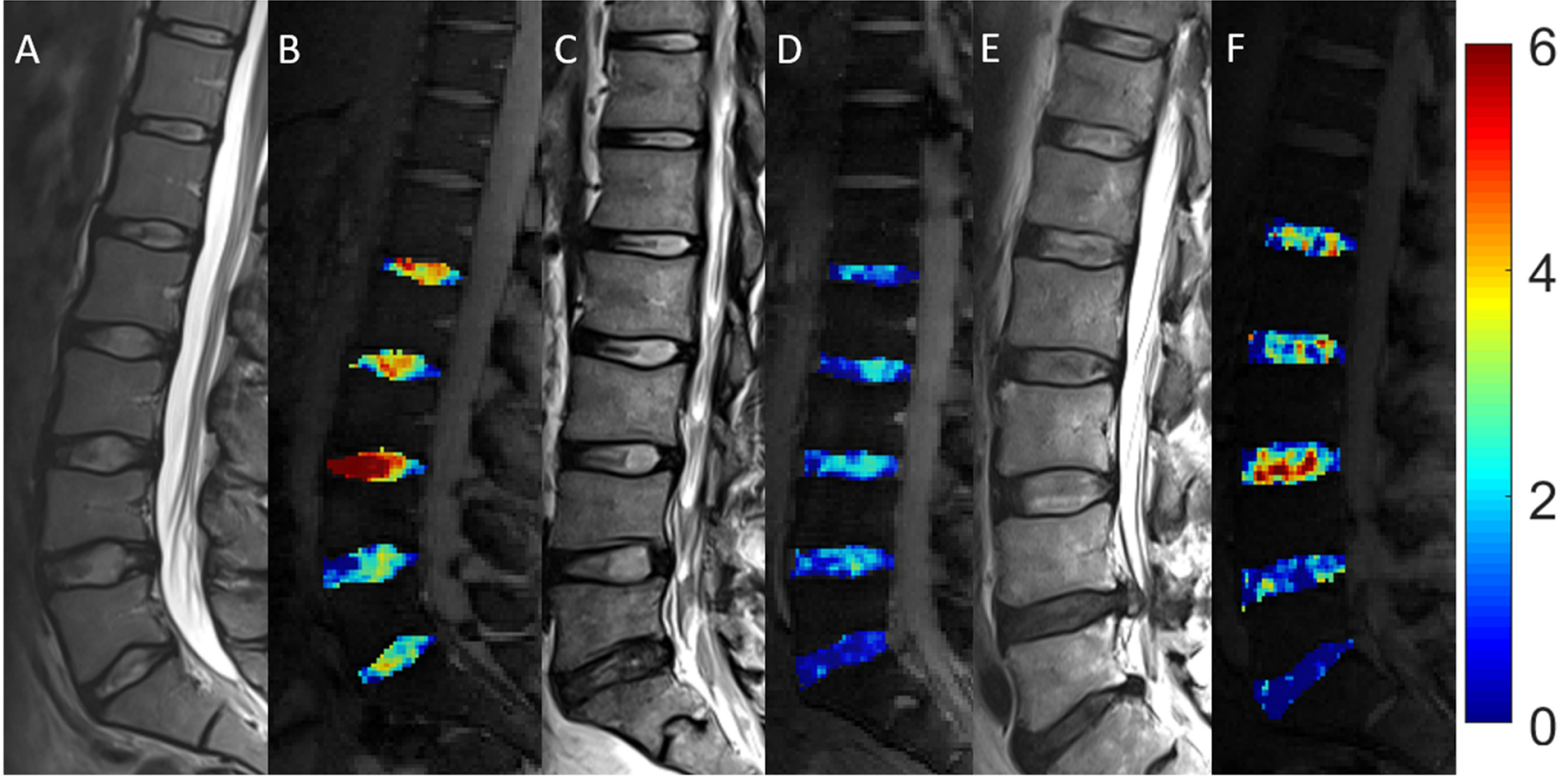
Morphologic and compositional imaging findings of lumbar intervertebral discs of an asymptomatic volunteer (A,B), a patient with nonspecific low back pain (C,D), and a patient with radiculopathy (E,F). (A,C,E): Sagittal T2-weighted (T2w) images show the absence of morphologic signs of relevant IVD degeneration (A), substantial dehydration at the L4/L5 segment (C) and the L5/S1 segment (C,E) accompanied by extrusion at the L4/L5 segment (E) (B,D,F) Sagittal glycosaminoglycan Chemical Exchange Saturation Transfer (gagCEST) images with overlaid color-coded maps to visualize the GAG contents of the IVD segments. Low GAG content is depicted in blue, and high GAG content is depicted in red. The unit of scale on the right is gagCEST effect in %. The lowest values are found in the patient with non-specific low back pain (nsLBP), while the highest values are seen in the asymptomatic volunteer. Reproduced with permission from Frenken et al., (57).
